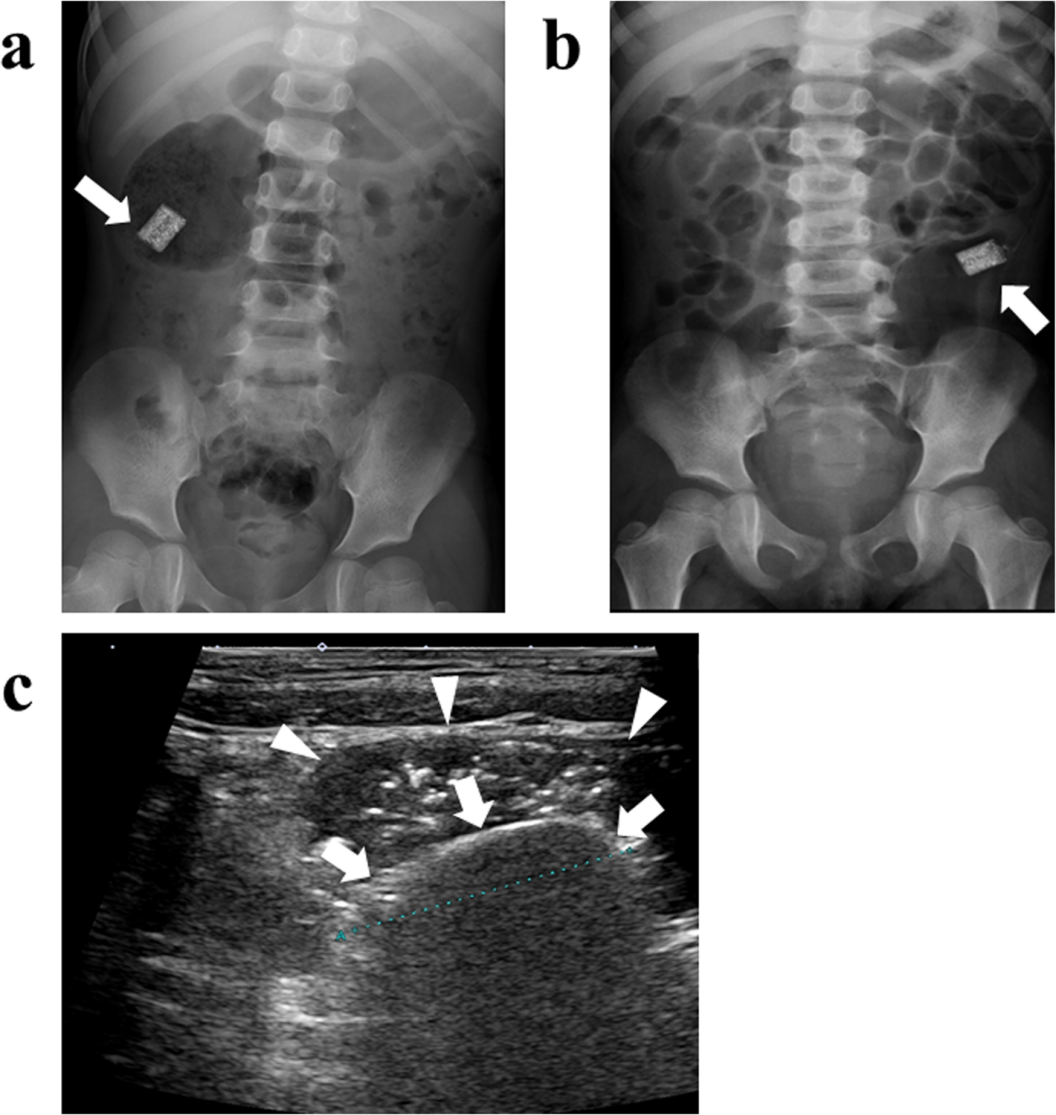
Patency capsule stagnation (arrow) at the dilated small intestinal tract. a shows the abdominal X-ray in the standing position; b shows the abdominal X-ray in the supine position; c shows stagnation of the patency capsule (arrow) at the dilated small intestinal tract (arrowhead)
